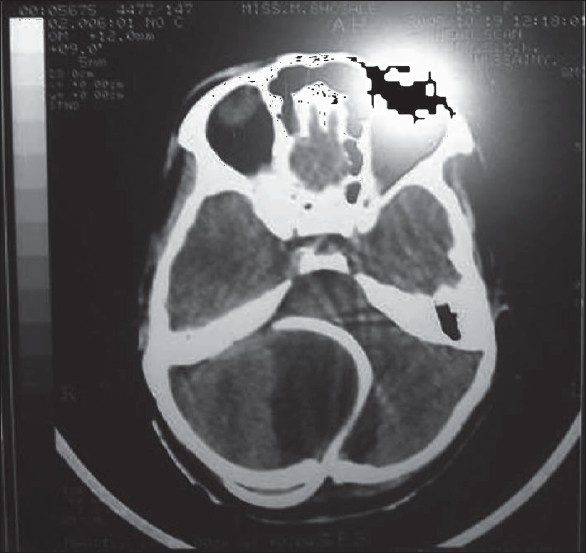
Post operative CT sacn with shunt *in situ*
